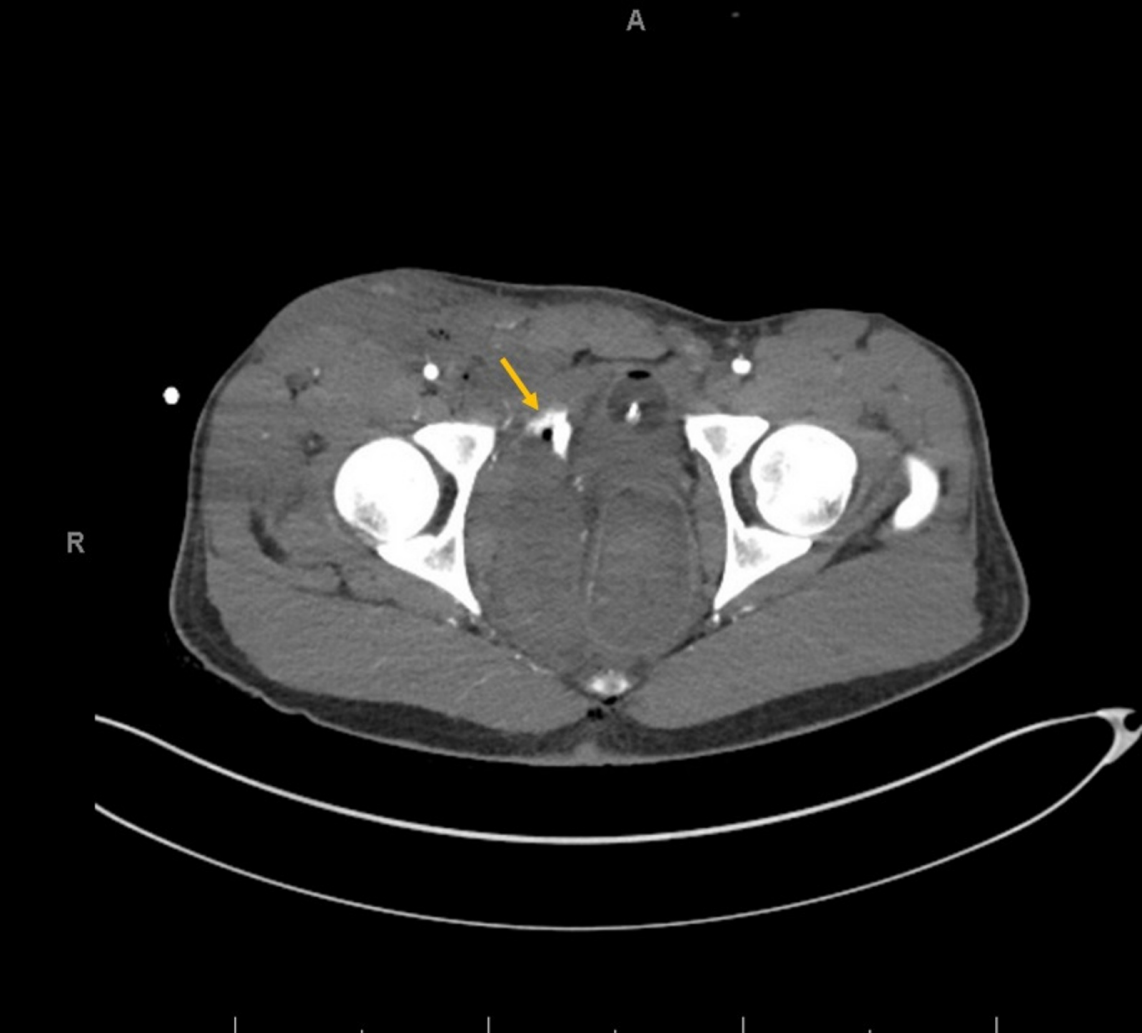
Computed tomography angiography (CTA) axial view Computed tomographic angiography (CTA) axial view of abdominal aorta and bilateral lower extremities demonstrating active extravasation of contrast (arrow) in the right perirectal region extending anteriorly along the right obturator internus displacing the prostate rectal vault and bladder.
